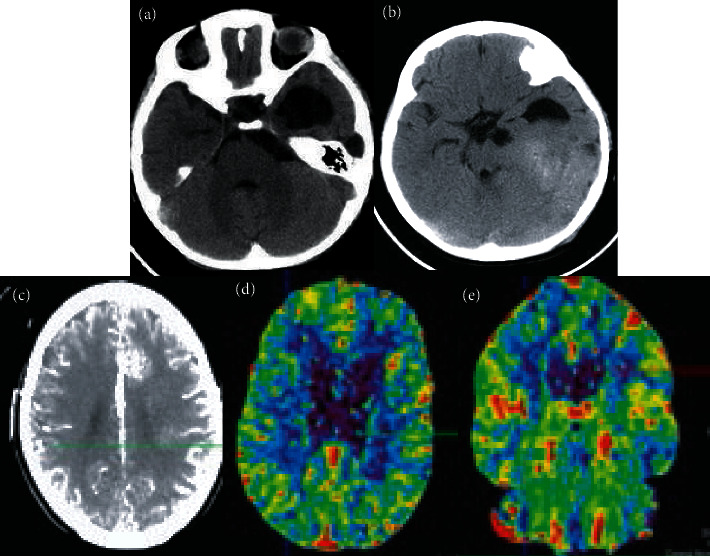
Imaging data of patients. (a)-(b) Ordinary CT. (c) Cranial enhanced CT. (d)-(e) CTPI.
